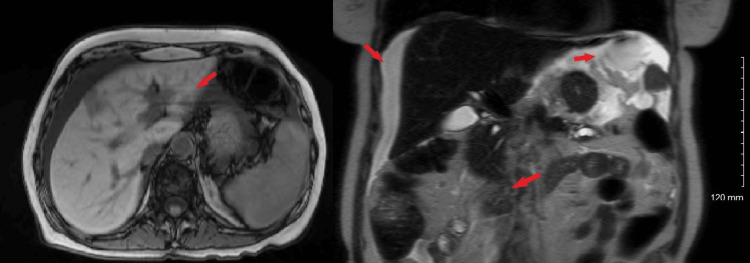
MRI of the abdomen with and without contrast These images demonstrate an ill-defined malignant neoplasm in the body of the pancreas, with distal pancreatic ductal dilatation (arrows). Additional findings include hepatic and omental metastases, mild ascites, abdominal lymphadenopathy, and left adrenal gland thickening, suggestive of metastatic disease. MRI: magnetic resonance imaging
